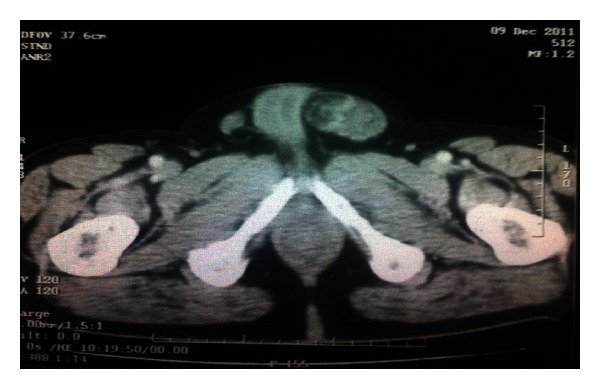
CT scan showing the left inguinal mass.
